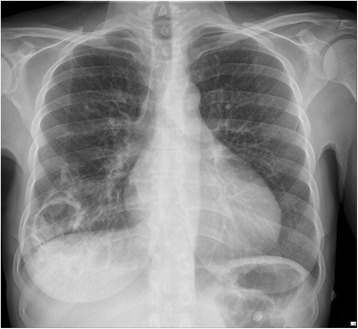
Chest X-ray showing a community-acquired pneumonia due to *A. pittii* with a large pulmonary cavity of the right lower lobe, at hospital admission
